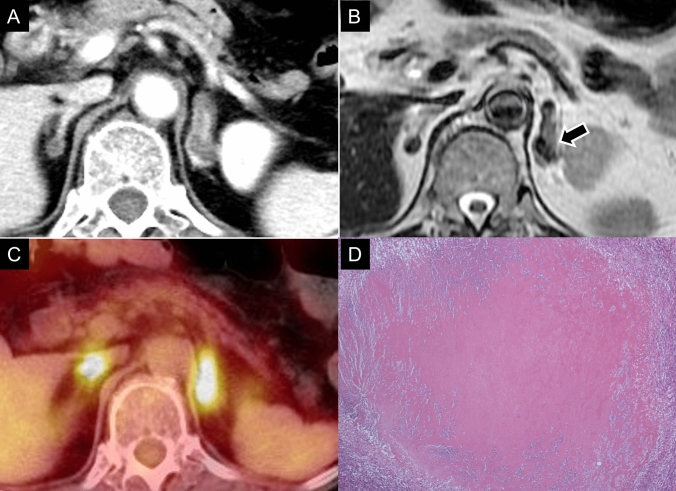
Adrenal tuberculosis. A 74-year-old female presented with an incidental adrenal mass. A Enhanced computed tomography (CT) showing bilateral adrenal masses with low attenuation. B T2-weighted magnetic resonance imaging showing low signal intensity in the lesions (arrow). C
